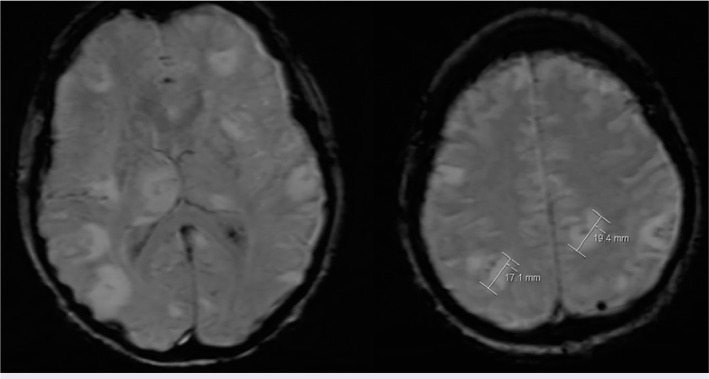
Multiple lesions are hyperintense on T2-weighted MRI with focal noduler after administration of contrast.
